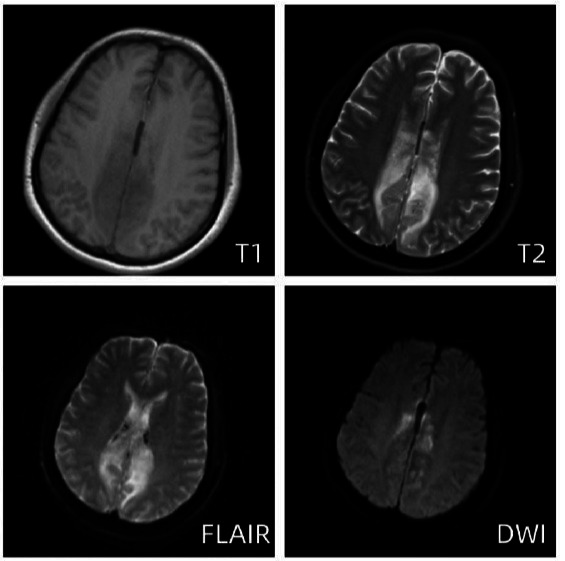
Brain MRI on day 5 after the onset of the disease. MRI showed that the T2 and FLAIR sequences of bilateral frontal-parietal paracentral and corpus callosum pressures showed markedly increased signal intensity in the cortex and subcortical white matter, suggesting vasogenic edema. DWI showed diffusion-restricted foci of bilateral parietal and corpus callosum pressures, suggestive of acute infarctions, while the remainder of the brain parenchyma exhibited no discernible abnormal signal patterns.
